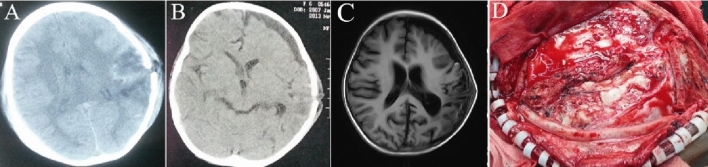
The imaging characteristics and intraoperative findings of Type III. (A) On the first day after injury, CT showed a linear fracture of the left temporoparietal bone and a cerebral contusion below the fracture line. (B) On the 19th day after the injury, CT showed a linear fracture of the left temporoparietal bone, the cerebral contusion below the fracture line was better than before, and local subdural effusion had formed. (C) On the 41st day after the injury, the MRI showed a fracture line herniation of the left temporoparietal brain tissue, local cystic changes and necrosis of the brain tissue, and local subdural effusion. (D) Extensive dural defects, contusion, local brain tissue contusion and necrosis were observed during the operation.
